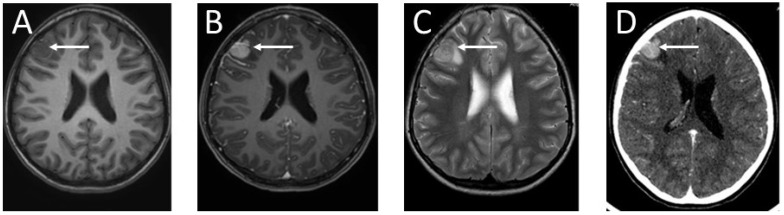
Imaging of the brain tumor: (A) T1-weighted MRI of the patient’s head without contrast enhancement—the brain tumor is visible as a hypointense lesion in contrast to the white matter, located in the right frontal lobe; (B) contrast-enhanced T1-weighted MRI—the mass in the right frontal region shows intense contrast enhancement; (C) T2-weighted head MRI—the tumor in the right frontal area is visible as a hyperintense lesion in contrast to the white matter; (D) CT diagnostic imaging after contrast enhancement—the tumor in the right frontal region is visible as a hyperdense lesion in contrast to the white matter of the brain. The arrows indicate the tumor location.
